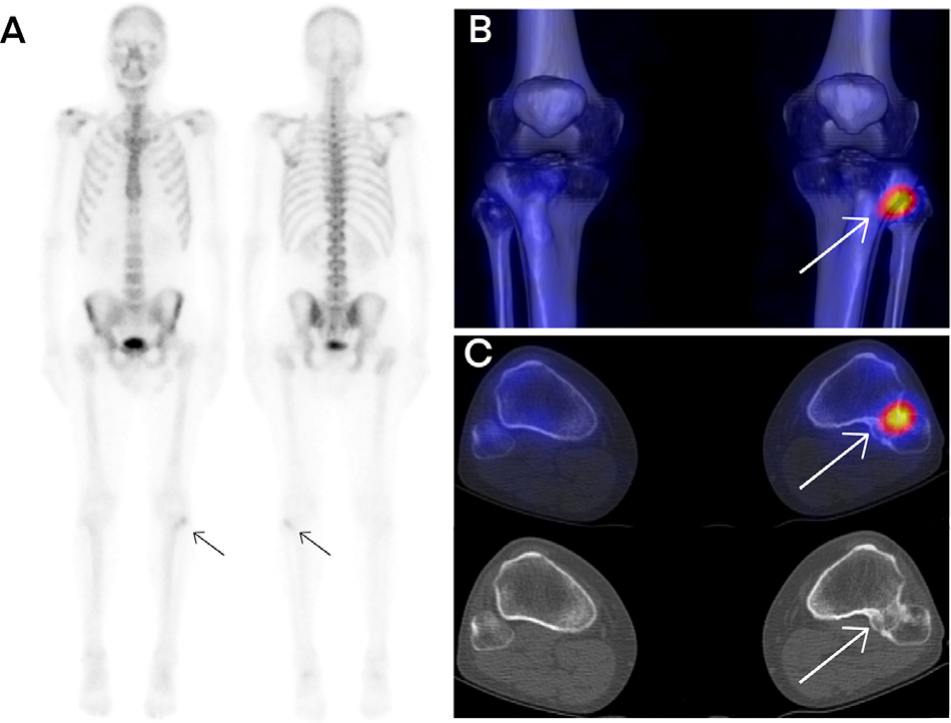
(A) Whole-body scintigraphy showing focal increased uptake at the proximal tibiofibular joint. (B) 3D SPECT/CT fusion and (C) 2D SPECT/CT images demonstrating bony bridging between the proximal tibia and fibula.
